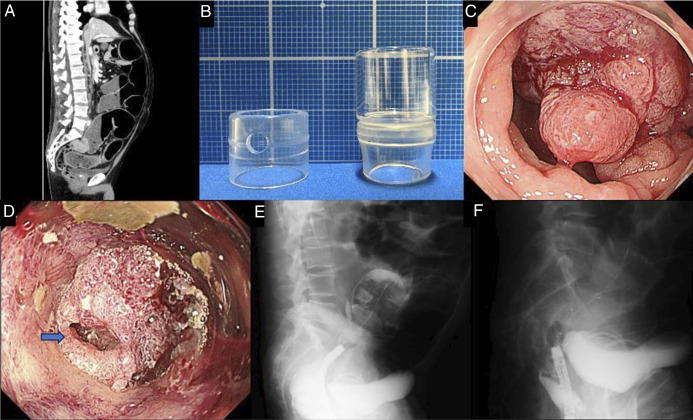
Clinical images of malignant large bowel obstruction because of rectal cancer and the endoscopic procedure using cap-assisted technique. (A) Pretreatment axial abdominal computed tomography image showing marked colonic dilatation proximal to the obstructive lesion in the rectum, suggestive of malignant large bowel obstruction. (B) Endoscopic caps used during the procedure. Left: short cap (4-mm protrusion; D-201-13404, Olympus, Tokyo, Japan); right: long cap (12-mm protrusion; MH-463, Olympus). (C) Endoscopic view of the anal side of the tumor using a short cap. The lumen is not identifiable. (D) Endoscopic view of the anal side of the tumor using a long cap. The lumen is more clearly visualized, and scope alignment is facilitated. Arrow indicates the lumen. (E) Fluoroscopic image after successful guidewire placement across the stricture. (F) Fluoroscopic image following deployment of a self-expandable metallic stent (SEMS), showing adequate expansion across the stenosis.
